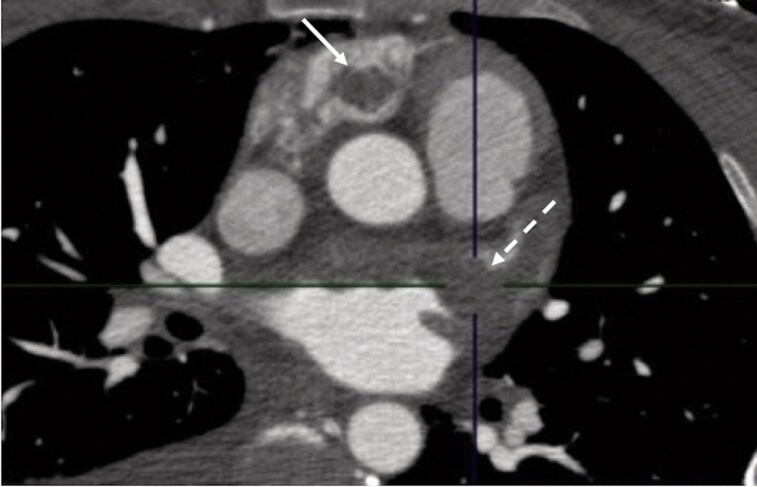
Contrast-enhanced CT. Solid arrow: thrombus in the right atrial appendage; dotted arrow: thrombus in the left atrial appendage.
